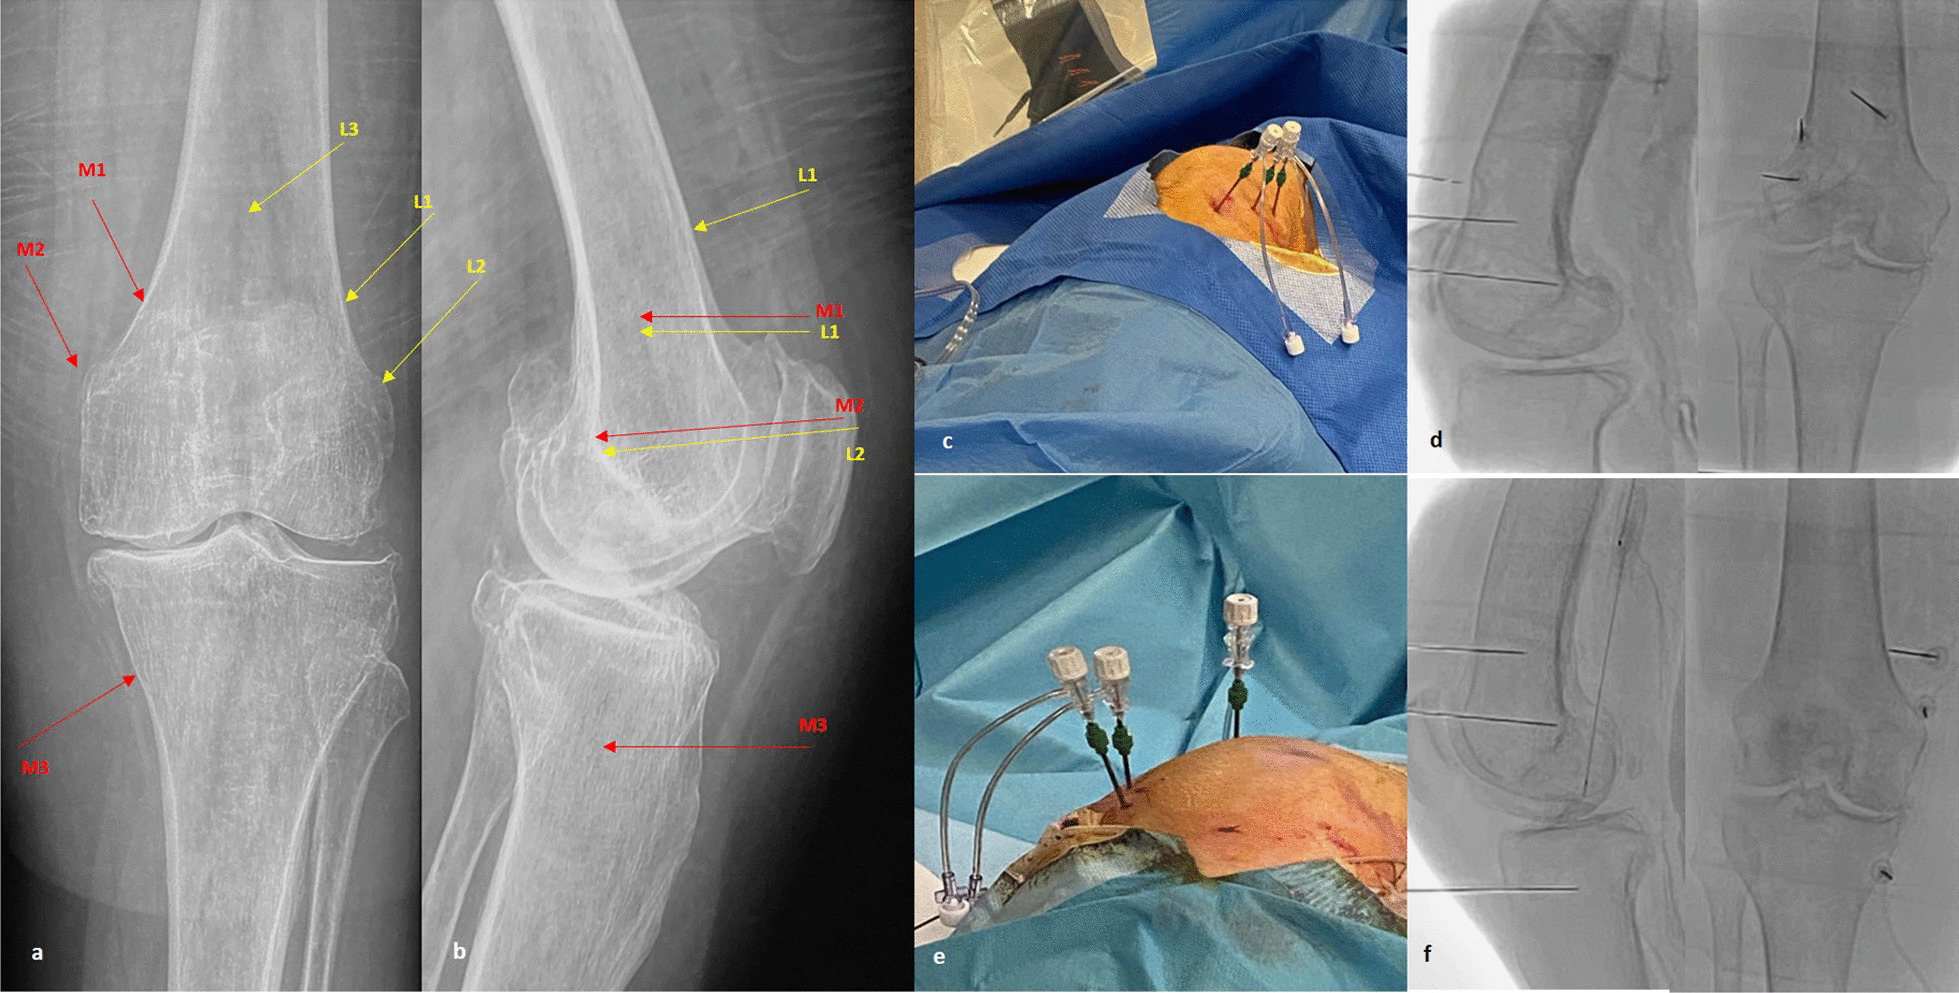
a, b Anteroposterior and lateral radiographs of an arthritic knee showing the intended target-points of the genicular nerves (M1, M2 = SMGN, M3 = IMGN, L1, L2 = SLGN, L3 = MRGN); c intraoperative picture of the needles in the lateral side just prior to ablation d anteroposterior and lateral fluoroscopic images of the exact location of the needles in the lateral side; e intraoperative picture of the needles in the medial side just prior to ablation; f anteroposterior and lateral fluoroscopic images of the exact location of the needles in the medial side
